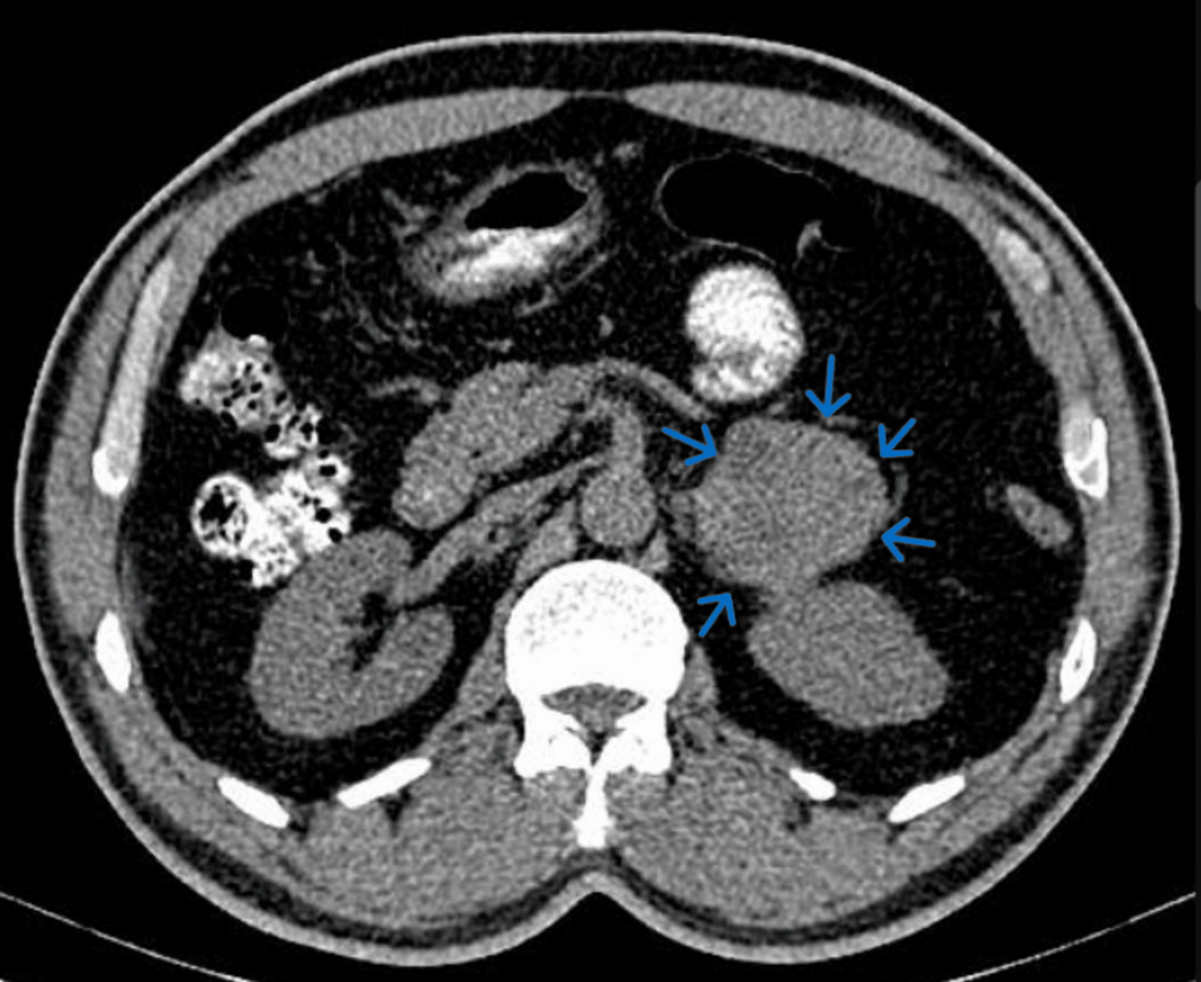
Abdominal CT scan image showing an adrenal gland mass (blue arrows) CT: Computed tomography
